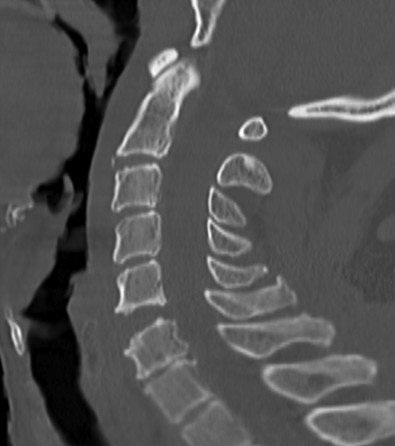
Sagittal cervical spine CT reconstruction-image at the local hospital shows subluxation at C5-C6 with disk-space diastasis demonstrating concern for extension-distraction injury.
